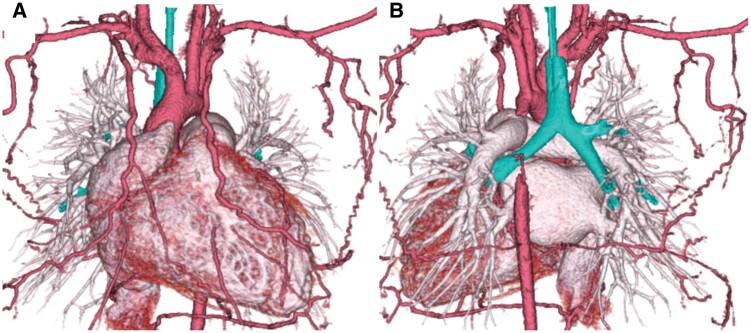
Preoperative computed tomography showing long-segment obstruction from the distal arch to the descending thoracic aorta. (A) Anterior view and (B) posterior view.
